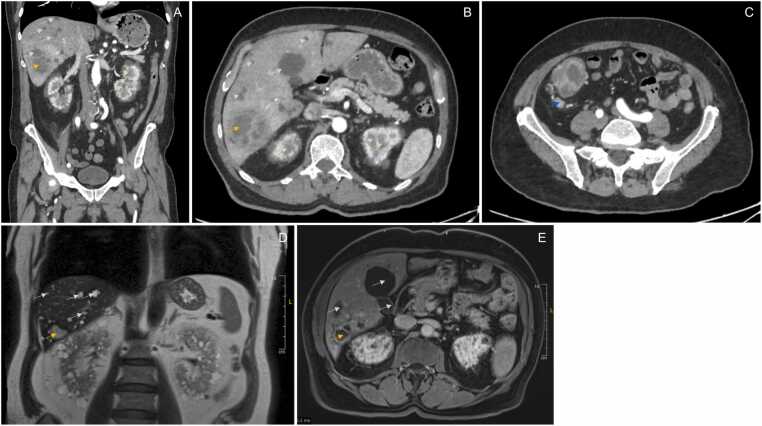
CT scan upon arrival (A) Frontal section after injection of iodinated contrast medium in the arterial phase showing innumerable hepatic and renal cysts (a few represented with green arrow) with poorly defined lesion formation at the hepatic apex (yellow arrow); (B) Axial section after injection of iodinated contrast medium in the arterial phase focusing on the hepatic amoebiasis abscess (yellow arrow); (C) Axial section after injection of iodinated contrast medium in the arterial phase showing the caecal pseudo-mass (blue arrow). MRI after 3-months follow-up (D) Frontal section showing innumerable hepatic and renal cysts (a few represented with green arrow) with hepatic abscess of 2,3 cm with T2-weighted intermediate signal (yellow arrow); (E) Axial section showing cocarde contrast of the hepatic abscess after gadolinium injection (yellow arrow)
